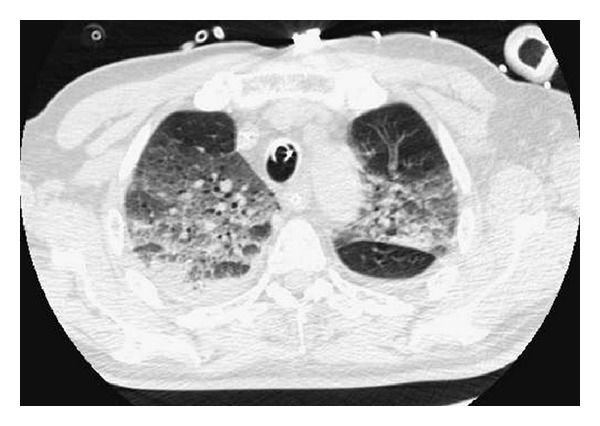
CT scan of the chest obtained on Day 1 showing diffuse bilateral alveolar infiltrate.
